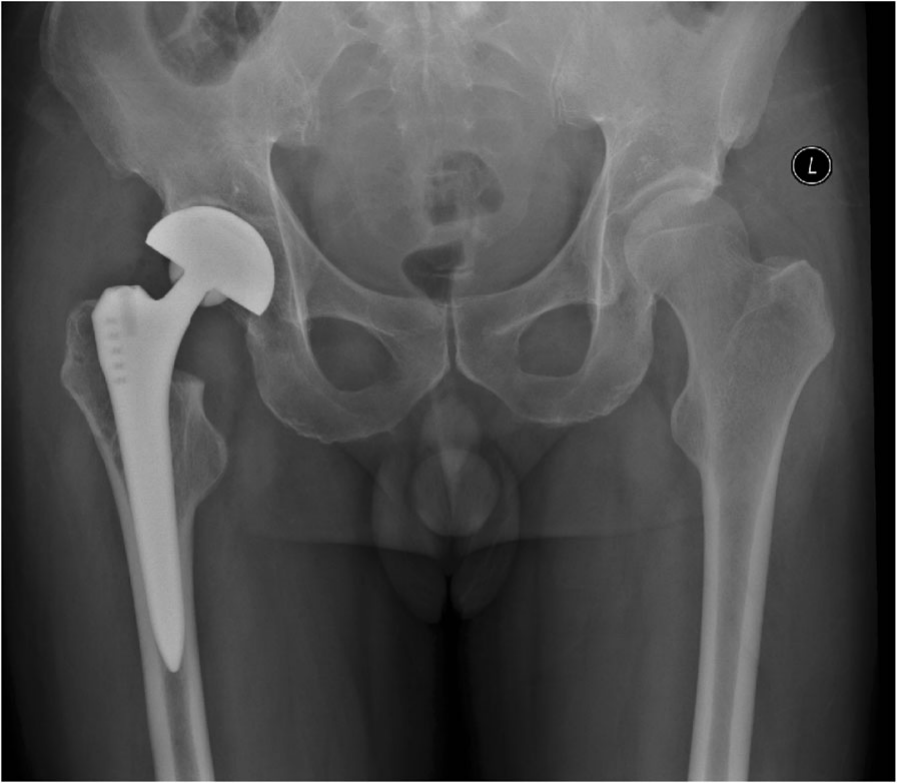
Anteroposterior radiograph of right cementless CoC total hip replacements
